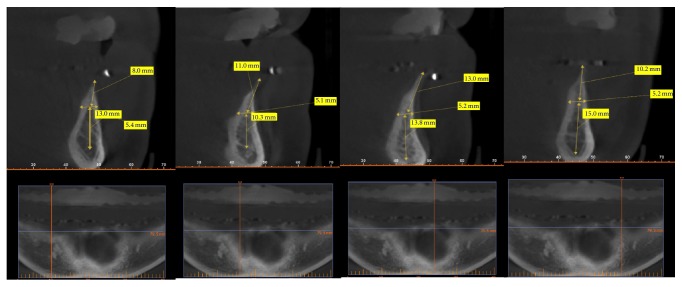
Cone beam computed tomography (CBCT) findings showing very thin coronal portion of the alveolar ridge.
